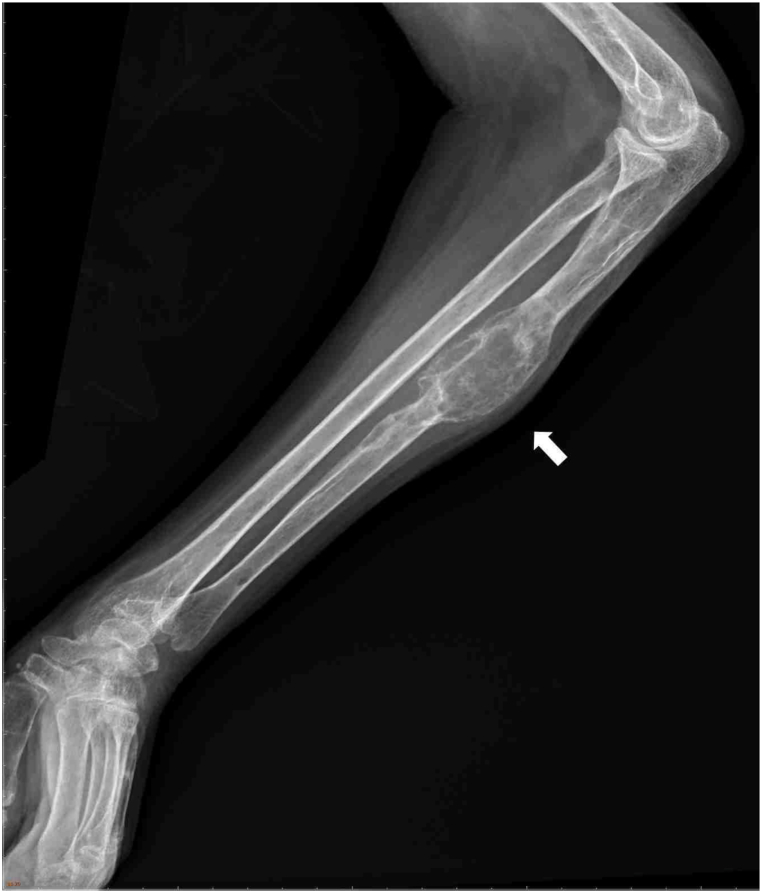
Bubbly-like cystic change and partial sclerotic margin at the left ulna midshaft metacarpal in X-ray.
